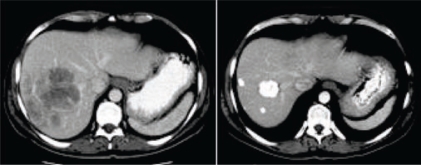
Patient with a partial response to i.a./i.v. chemotherapy. (a) pre-treatment CT shows a huge lesion into the right lobe; (b) post-treatment CT confirms lesion reduction, detecting some calcifications within the treated lesions
